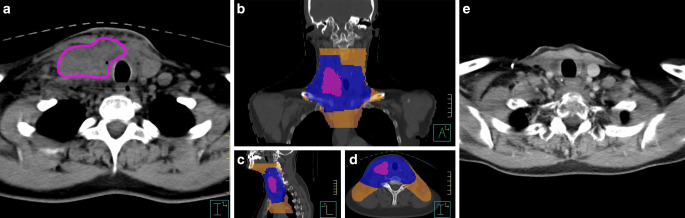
Patient 1 (Table 2). a Macroscopic residual tumor after surgery and before radiochemotherapy. b Planning target volume (*orange*): single fraction dose: 1.8 Gy. c Cumulative prescribed dose: 50.4 Gy and boost volume (*blue*): single fraction dose: 1.8 Gy. d Cumulative prescribed dose: 64.8 Gy, macroscopic residual tumor volume (*pink*). e Last follow-up computed tomography (CT) scan: no evidence of disease
